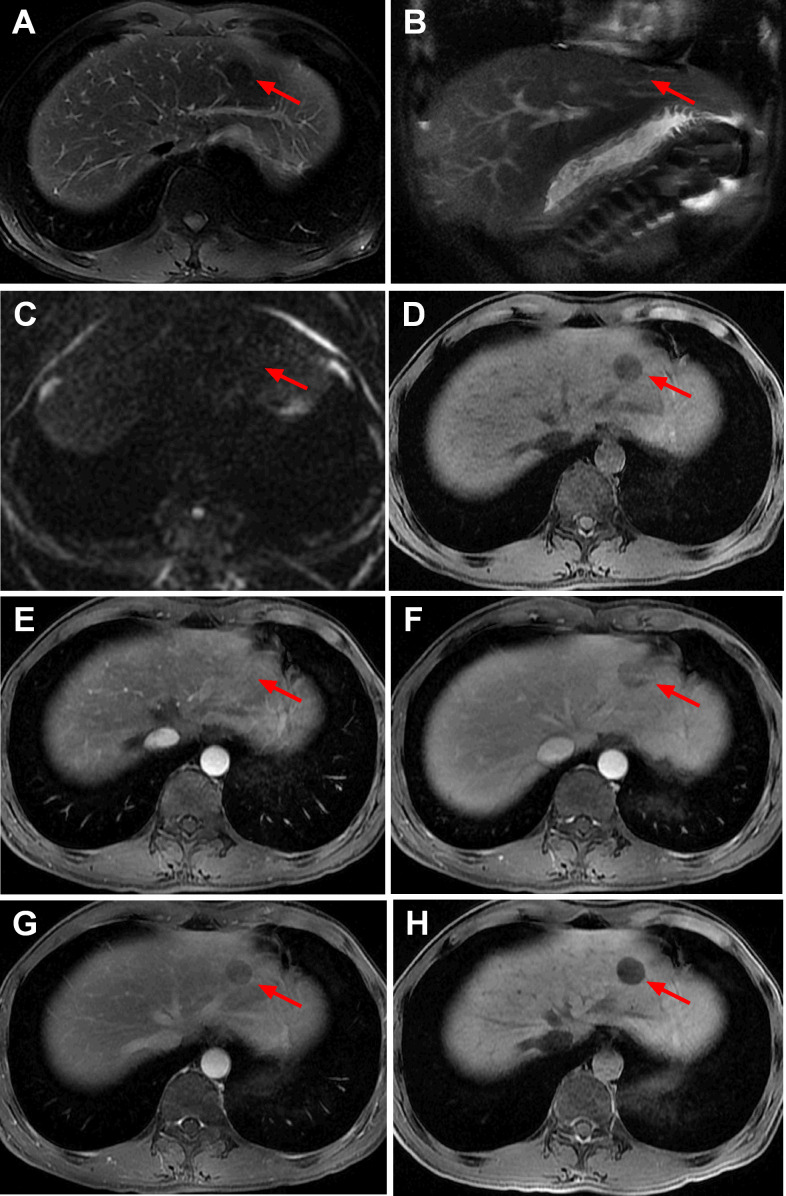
MRI images of IHS in case two. The lesion in segment II had a low signal on T2WI-FS (A, arrow) and was located in the peritoneal folding area between the diaphragm, falciform ligament, and left hepatic capsule (B, arrow). The lesion was relatively isointense on DWI (C, arrow) and hypointense on T1WI (D, arrow) with a clear boundary. After the injection of Gd-BOPTA, the lesion exhibited isointense enhancement in the arterial phase (E, arrow), hypointensity in the portal vein phase (F, arrow) and delayed phase (G, arrow), and an enhancement defect in the hepatobiliary phase after a delay of 2 h (H, arrow).
